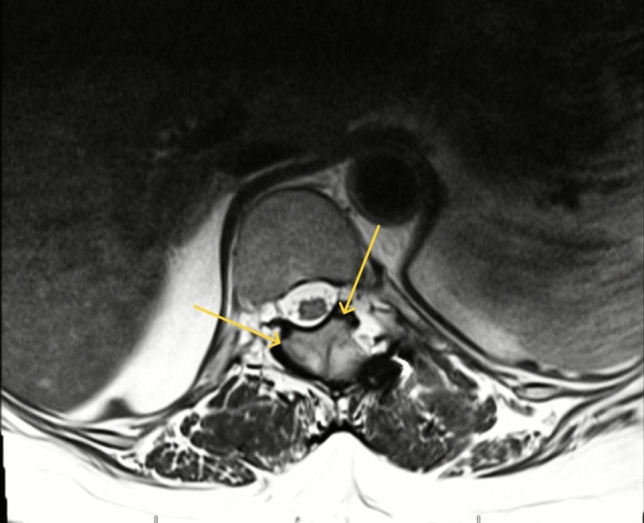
MRI demonstrating stenosis of the lumbar spinal cord
